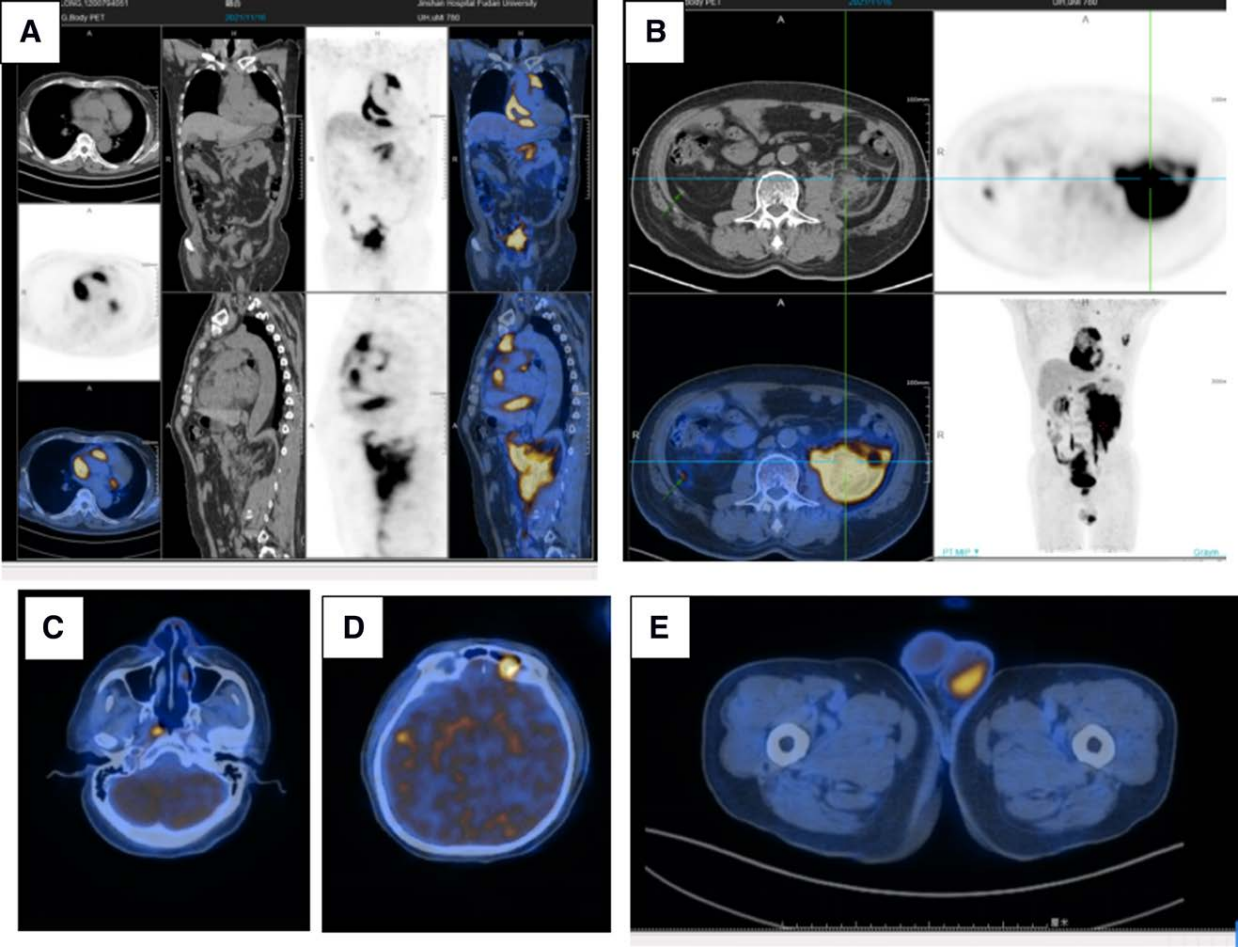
PET-CT scan images. (A–B). FDG uptake is increased in multiple areas of the body. (C). Abnormally elevated FDG uptake in the left orbital cavity; (D). Abnormally elevated FDG uptake in the left orbital cavity; (E). Abnormally elevated FDG uptake in the left testicle.
